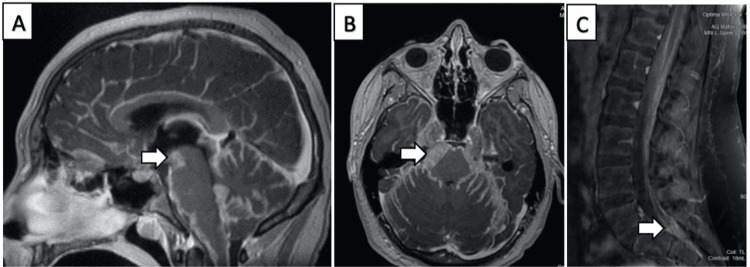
Brain MRI (A) Mid-sagittal T1 MRI of the brain with contrast enhancement demonstrates extensive “sugar coating” of the cerebral surface especially the cerebellum and brainstem.  Arrow points to bulky disease within the ambient cistern. (B) Corresponding axial T1 MRI of the brain with contrast showing extensive disseminated tumor surrounding the pons (arrow) and cerebellum. (C) Mid-sagittal T1 MRI of the lumbar spine with contrast showing “drop” metastasis at the end of the thecal sac (arrow) which was explored during surgery.
